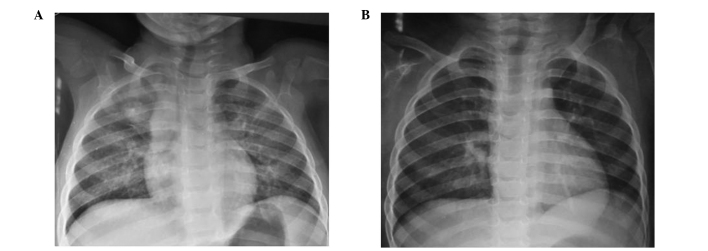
Chest X-rays of a 1.5-year-old female with a tuberculin skin test induration of 10 mm (BCG-vaccinated), (A) during diagnosis of pulmonary tuberculosis (TB) and (B) following treatment for pulmonary TB disease. The father was identified as a smear-positive case of pulmonary TB, and the child was diagnosed with active pulmonary TB after 120 days of TB-PT. Bilateral infiltrations, a pulmonary nodule at the right upper zone and a fracture of the clavicula were identified on the chest X-ray.
